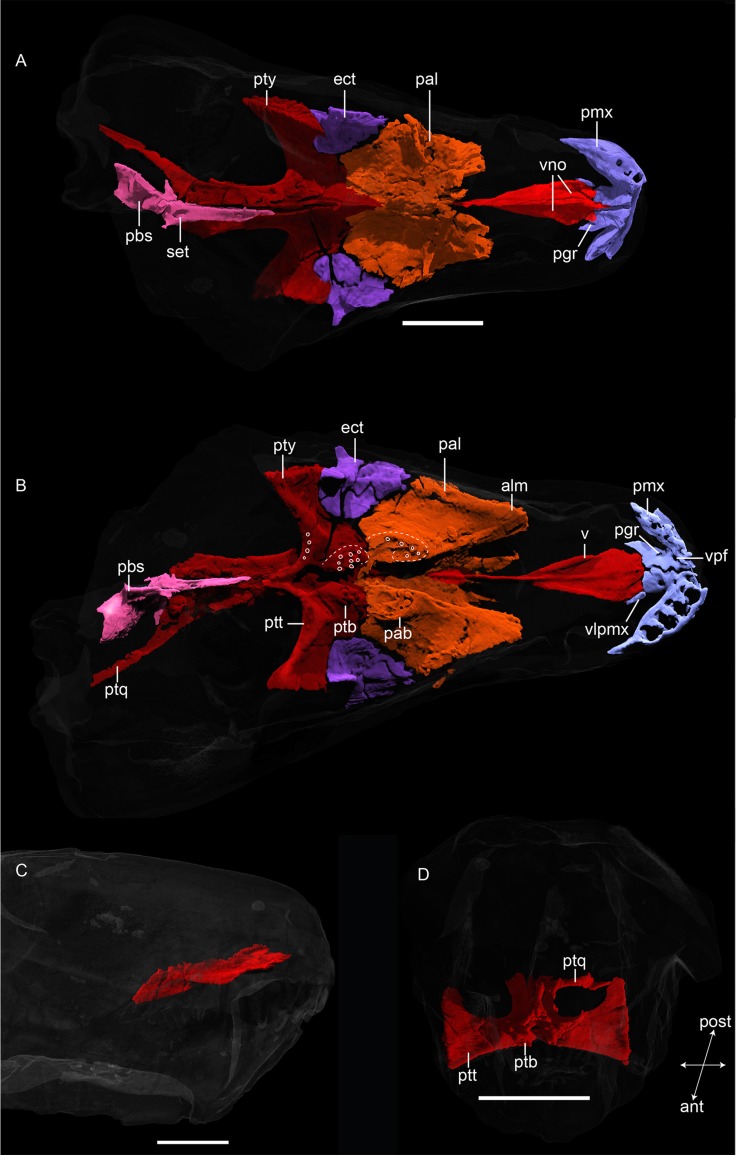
Neutron CT reconstruction of MB.R.999: (A) dorsal and (B) ventral palatal morphology, (C) vomer from right lateral, (D) pterygoid from anterior. White circles in (B) indicate position of teeth. Abbreviations: ect, ectopterygoid; pab, bosses of the palatine; pal, palatine; pbs, parabasisphenoid; pgr, premaxillary groove; pmx, premaxilla, ptb, bosses of the pterygoid; ptq, quadrate ramus of the pterygoid; ptt, transverse process of the pterygoid; pty, pterygoid; set, sella turcica; v, vomer; vno, hypothetical position of vomeronasal organ; vpf: ventral premaxillary foramen; vlpmx, ventral lamina of the premaxilla. Scale bars = 2 cm.
